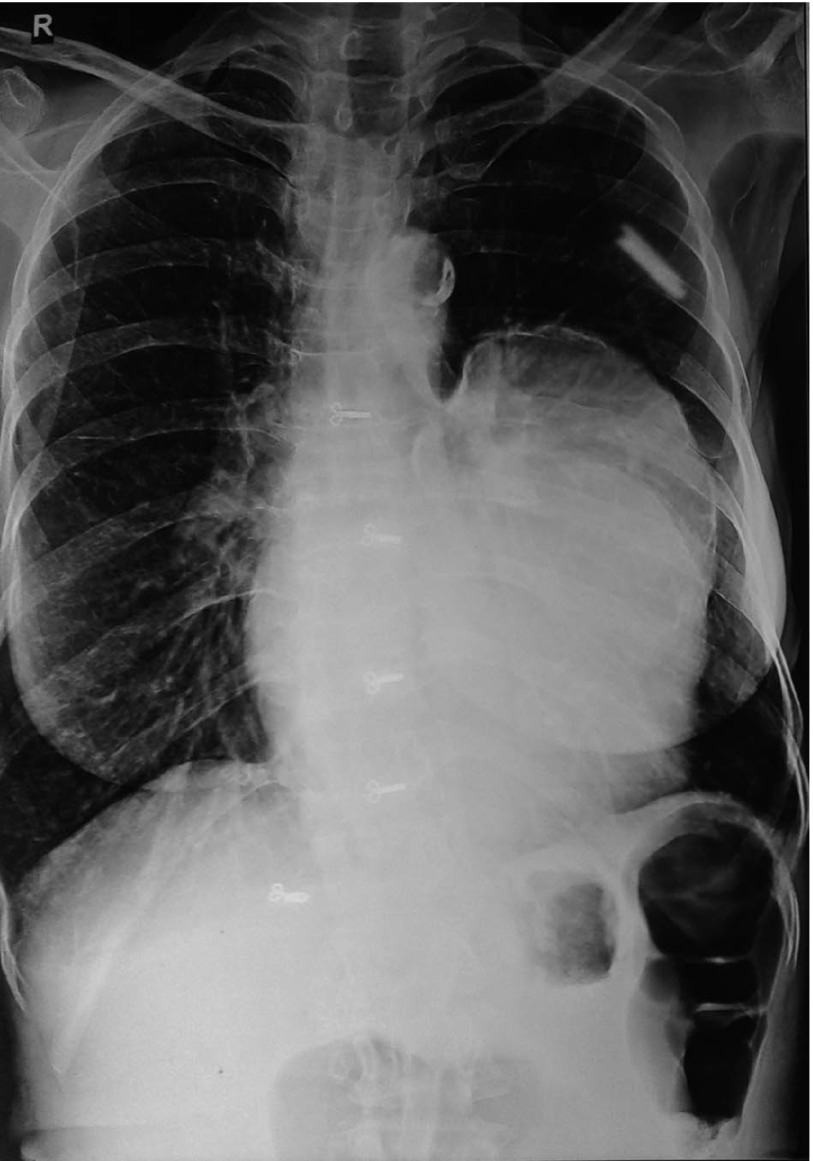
Chest X-Ray Showing Bulging of Left Heart Border
